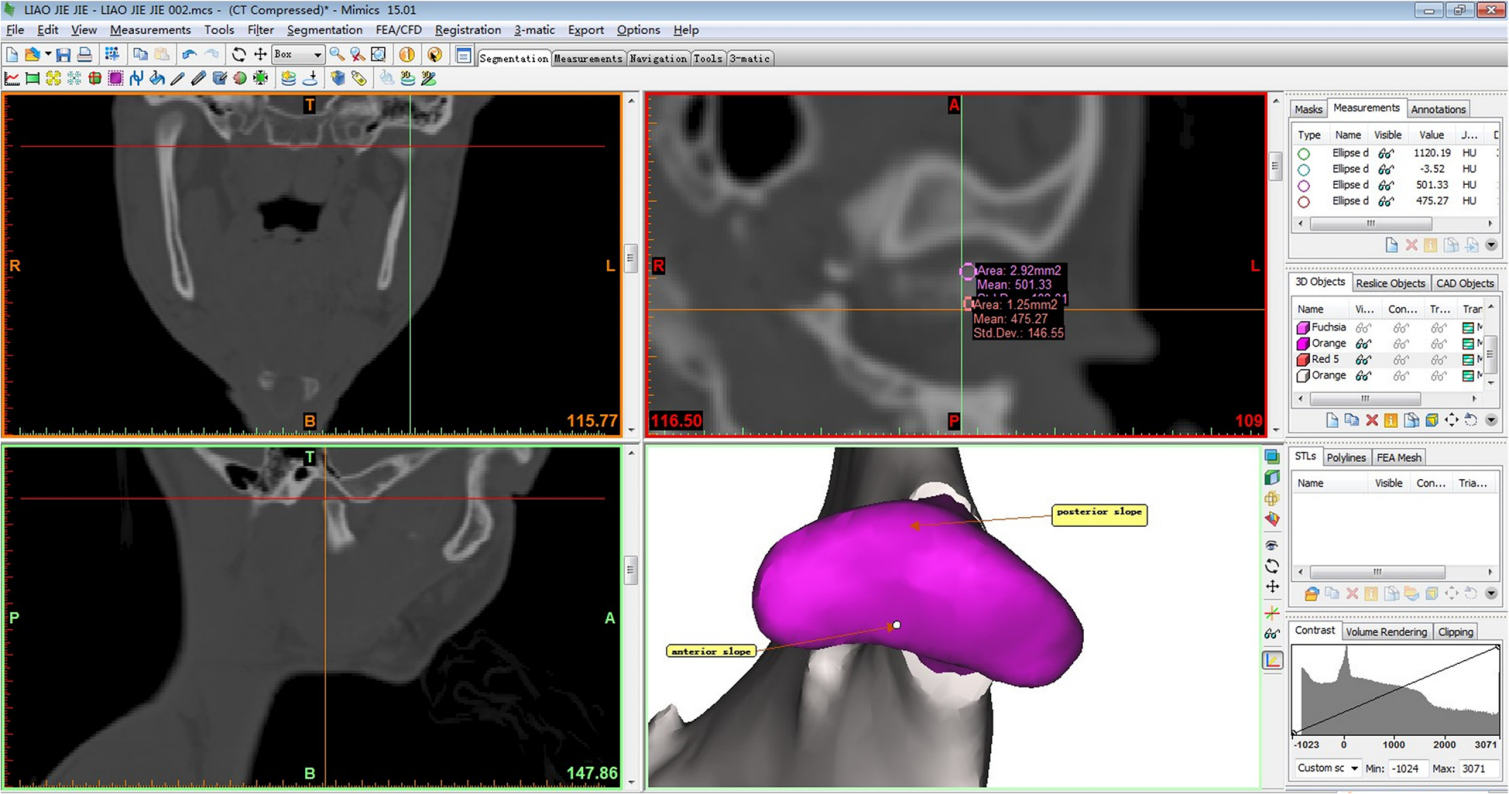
Bone mineral density (BMD) measurement of anterior and posterior slope of condyle. HU measurement made in CT image using MIMICS software.
